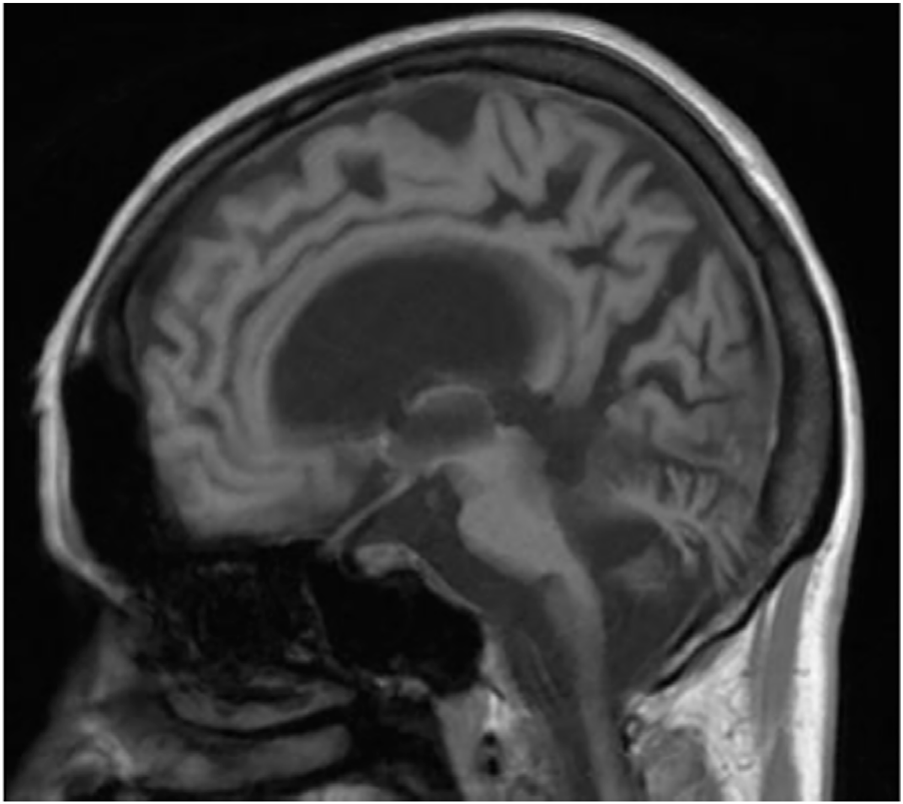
MRI—massive cortical and subcortical atrophy Sagittal section.
